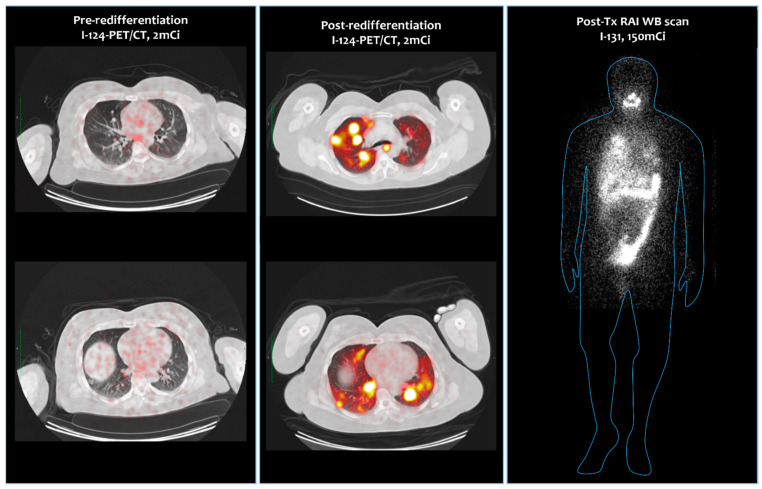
A successful redifferentiation of a BRAF (+) RAI-indifferent metastatic thyroid cancer with combined BRAF and MEK inhibition treatment. The first column of images on the left is the representative views of I-124 PET/CT obtained prior to redifferentiation treatment. The column in the middle demonstrates the views of I-124 PET/CT obtained after the redifferentiation treatment. The last column on the right is the post-treatment whole-body scan with 150 mCi activity (MCRC series).
